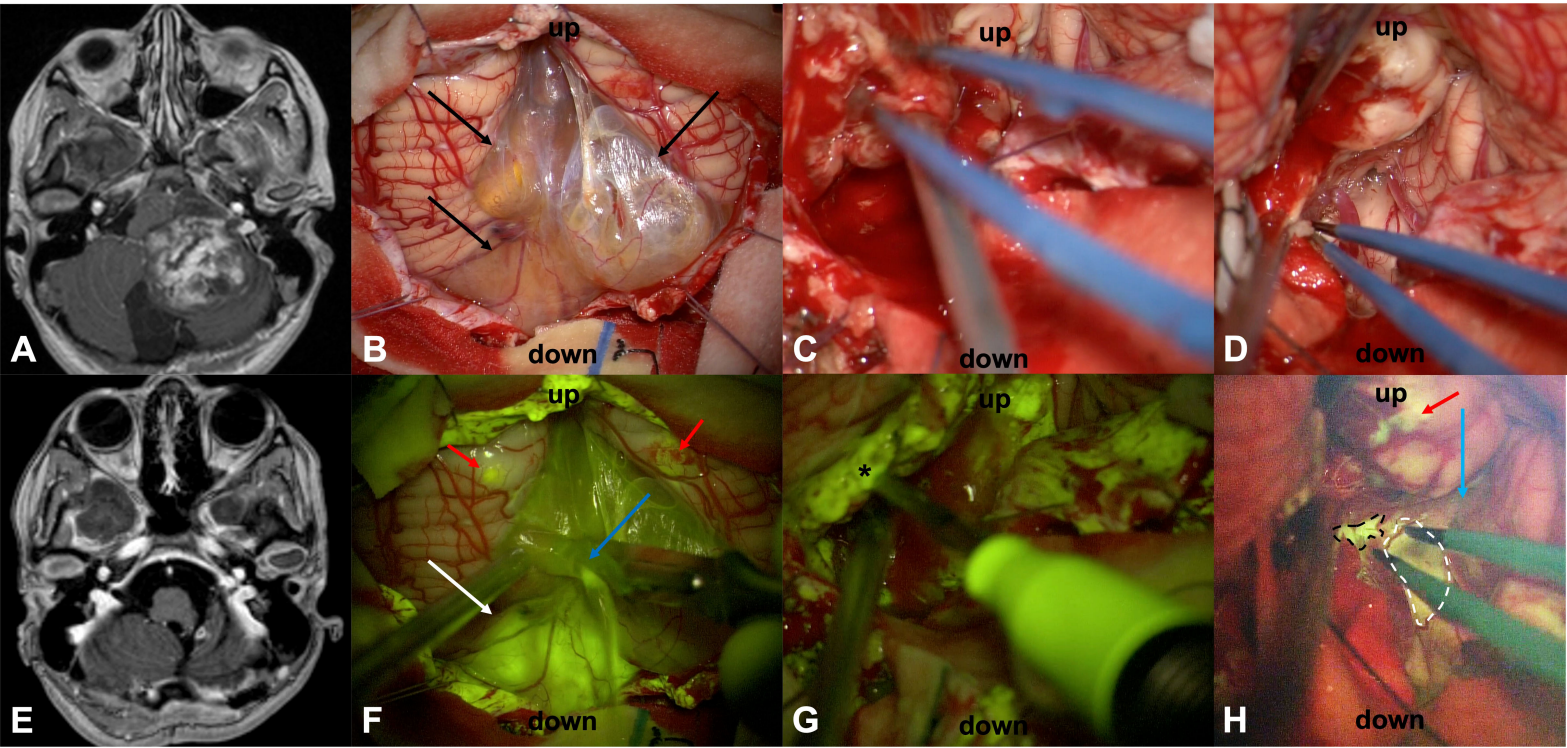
Preoperative T1 with contrast axial scan (A) in a 14-year-old female (patient n.20) shows a large posterior cranial fossa pilocytic astrocytoma with a subtotal resection, as detectable by postoperative post-contrast T1 MRI in (E). Surgery was performed in the sitting position. After dural opening, it was possible to appreciate a partially cystic lesion (arrows in (B)) that was better highlighted after activation of the YELLOW 560 filter (F), as a multilobulated cyst with a bright fluid (blue arrow) and an inferior vermis nodular component with an intense fluorescein enhancement (white arrow). Two small cortical areas of unspecific enhancement due to cerebellar manipulation during the initial phase of dural opening were visible as fluorescent spots (red arrows in (F)): this could happen because the damage to the cortex has been performed closer to fluorescein i.v. injection when a significant amount of fluorescein molecules is still present in blood circulation. The tumor was removed with a cavitron ultrasound aspirator, showing a bleeding, friable tissue (C) with inhomogeneous fluorescein enhancement after YELLOW 560 activation (asterisk in (G)). SF resulted particularly important to discriminate pathological fluorescent tissue (dotted lines) from normal peritumoral parenchyma (blue arrow) at tumor borders (D–H): minimal fluorescent tissue was left where the infiltration of the left lateral recess (black dotted line) and, less intensely, on the left part of the floor of the IV ventricle (white dotted line) made surgical resection hazardous for neurological functioning. The fact that the same minimal area of infiltration was not visible at postoperative MRI is related to the molecular weight of fluorescein, which is slightly lower than that of the contrast medium, that allows for a better delineation of BBB damage even in areas where contrast enhancement was not present. The unspecific enhancement due to cerebellar surgical manipulation at the beginning of the surgery was still visible with the YELLOW 560 filter activated (red arrow in H).
